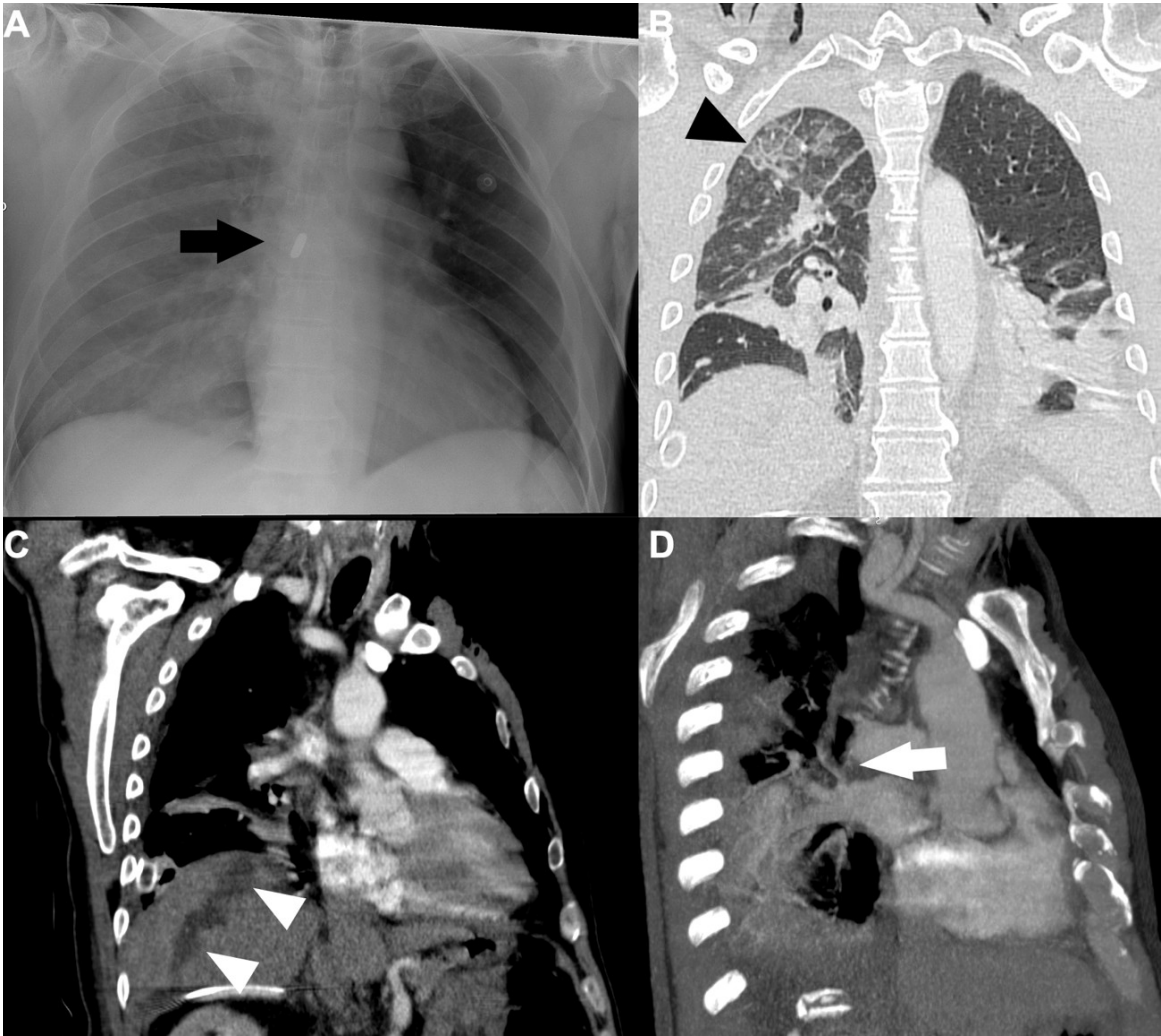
X-ray image (A) of a patient with a gunshot wound with the bullet lodged
